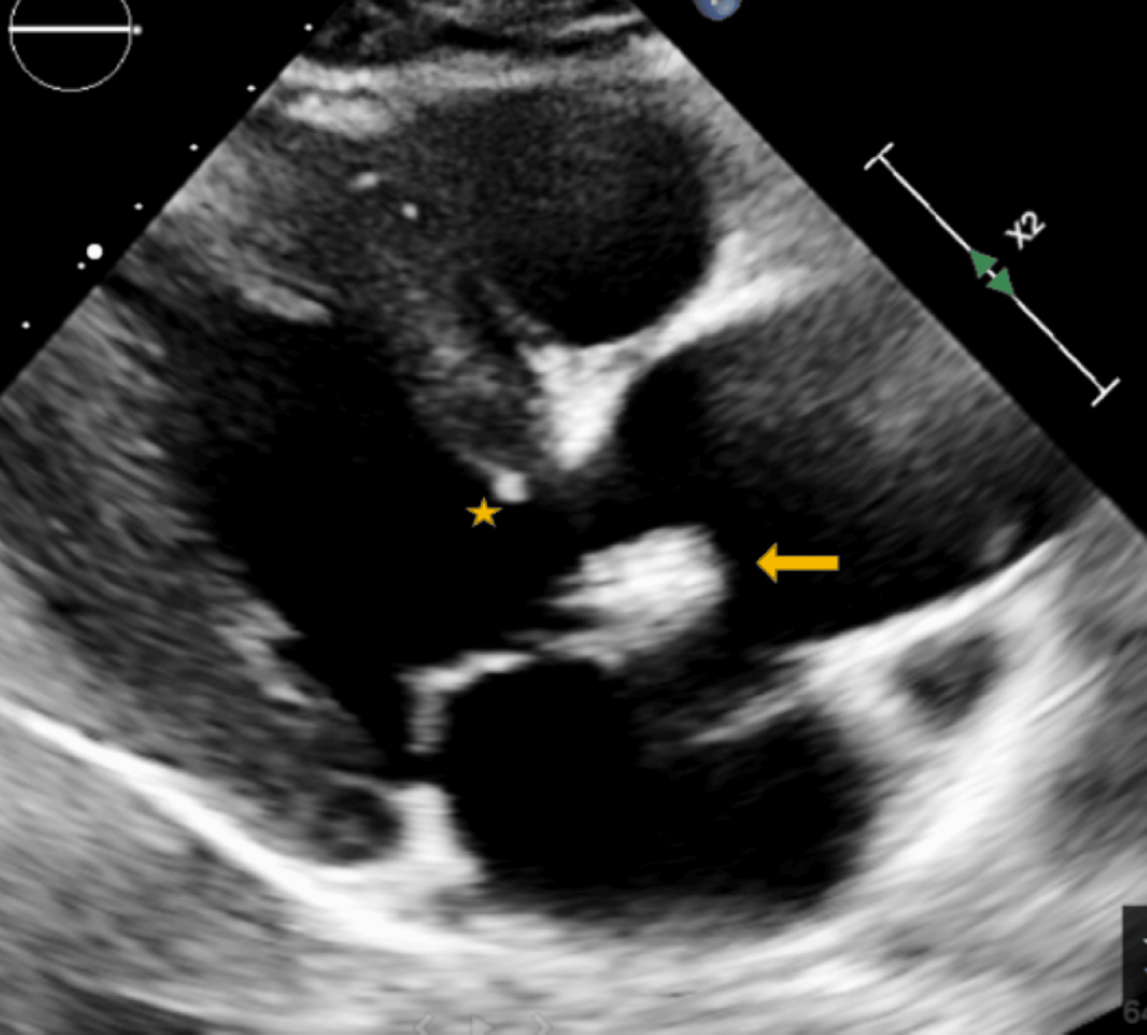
Parasternal long-axis TTE view demonstrating a discrete subaortic membrane (asterisk) located just below the aortic valve, along with focal calcification of the left coronary cusp (arrow). These findings contribute to subvalvular obstruction and structural valvular abnormalities noted on imaging X2 denotes a two-fold zoom applied by the echocardiography machine, indicating image magnification rather than an anatomical distance measurement. TTE: transthoracic echocardiogram
